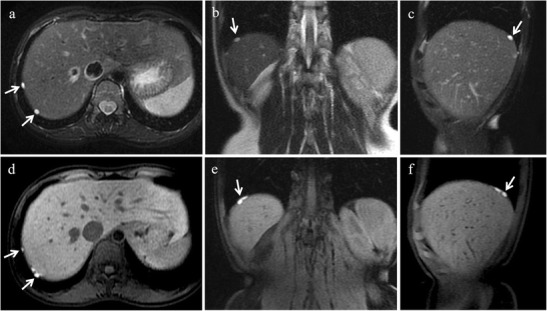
Diaphragmatic endometriosis in a 28-year-old woman with right-sided basithoracic chest pain associated with menses, who underwent a previous surgical intervention for endometrioma of the left ovary. (a) Axial, (b) coronal, (c) sagittal T2-weighted images, and (d) axial, (e) coronal and (f) sagittal fat-suppressed T1-weighted images show right-sided hyperintense nodular diaphragmatic implants (white arrows). The lesions are better depicted on fat-suppressed T1-weighted sequences
